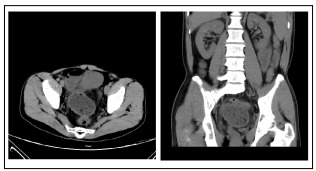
Abdominal and pelvic computed tomography examination: A large cystic mass with a clear boundary can be seen.
